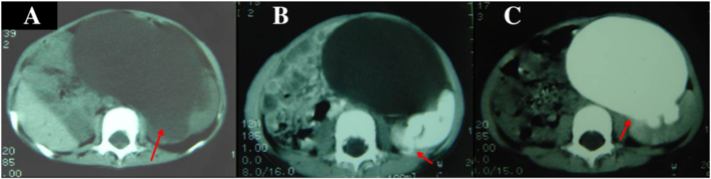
Case 4. CT scans obtained in a 2.5-year-old girl with left duplex ureters and congenital megalo-ureter. Non-enhanced scan obtained at the level of the inferior-middle pole of the left kidney shows a huge round-like area of low density (*red arrow*) (a). Contrast-enhanced scans were obtained at the same level as in (a), this area showed no enhancement on (b) the 26-h scan, and the tortuous contrast agent can be seen retroperitoneally (*red arrow*) (b). The huge round-like area of low density was intensified on the 48-h scan (*red arrow*) (c)
